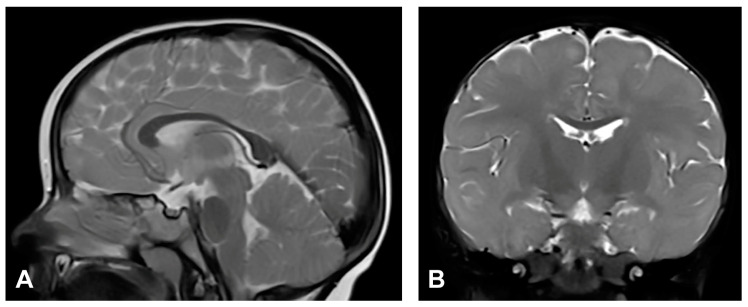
(Case 5) (A) Sagittal and (B) coronal T2W magnetic resonance images at the age of two without pathological findings.
